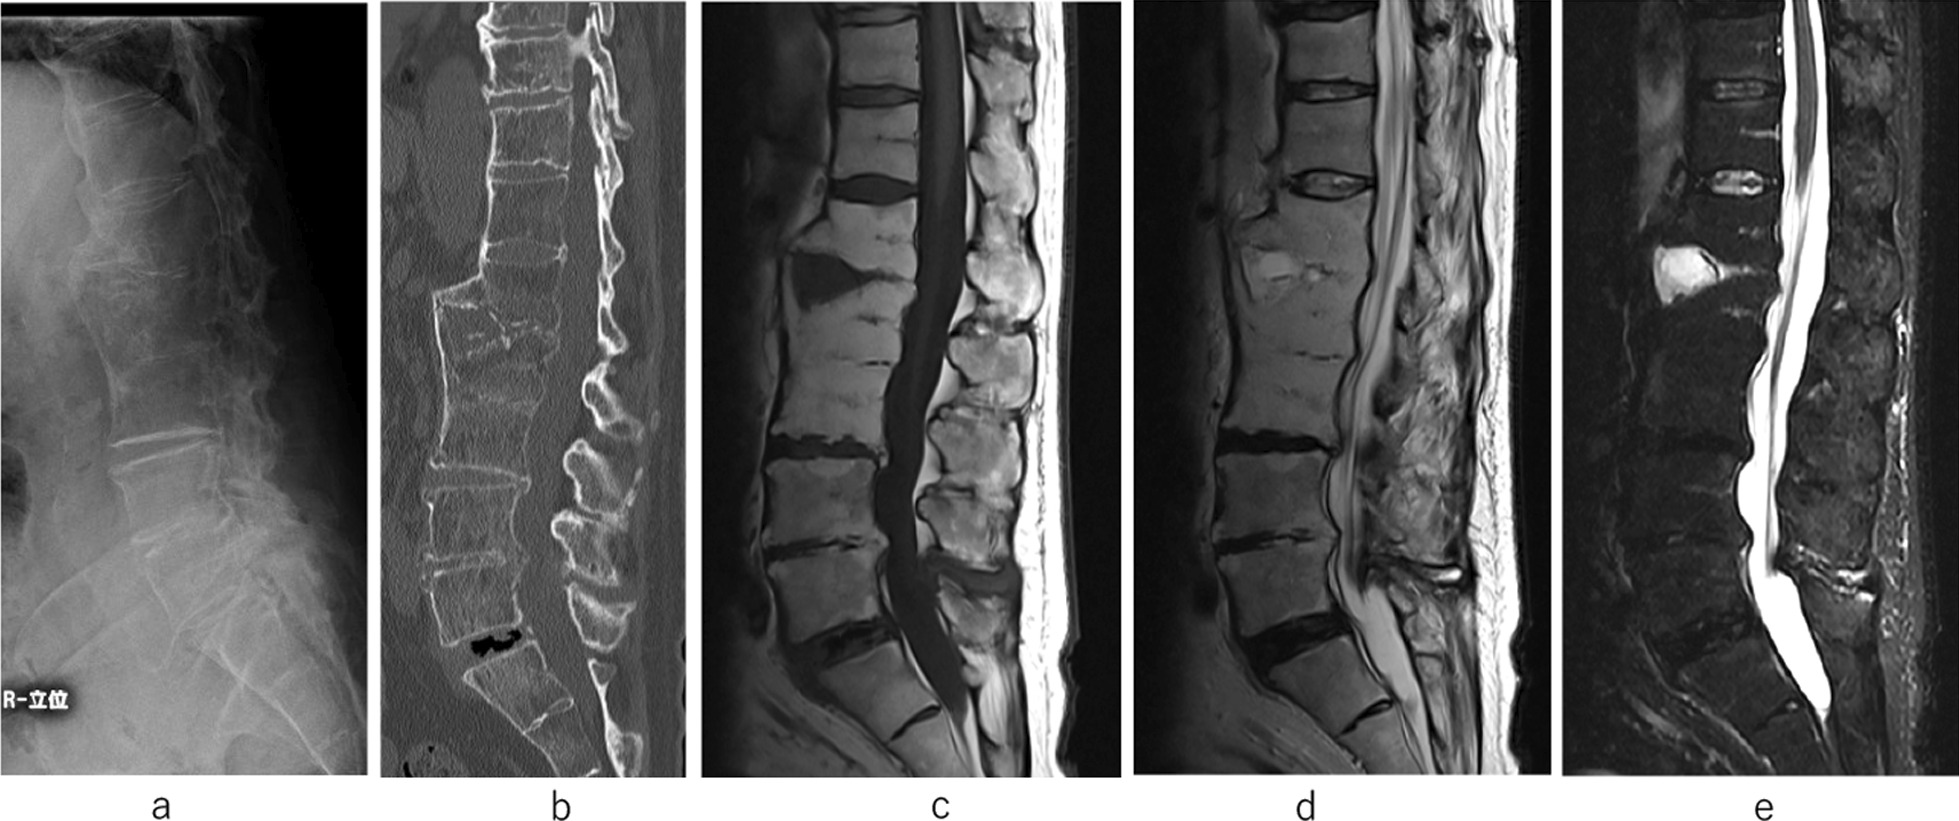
Plain X-ray imaging and computed tomography after screw removal. computed tomography showing bone regeneration at L1 and L2 at 1 year after surgery (a, b). MRI shows no bone marrow edema or bone cyst in the L1–2 disc space (c–e)
